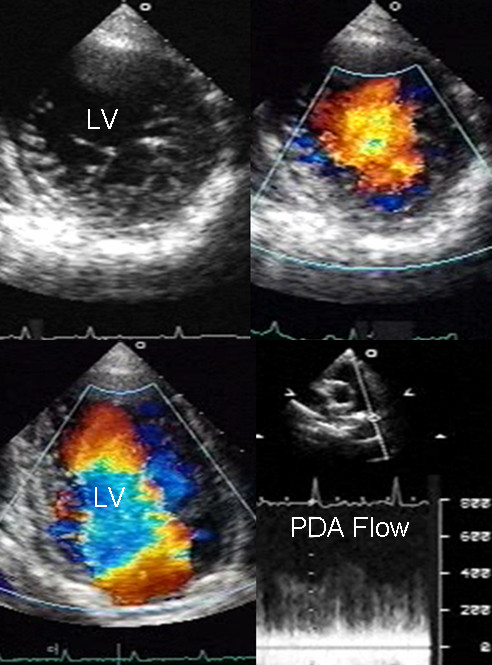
Transthoracic two-dimensional study with color and continuous wave Doppler shows left ventricular noncompaction associated with patent ductus arteriosus (PDA). Trabeculae and deep recesses with penetration of color can be observed in the left ventricle. Continuous wave Doppler from a suprasternal approach at the level of the great vessels registers systolic-diastolic flow through the ductus arteriosus. Others abbreviations as before.
